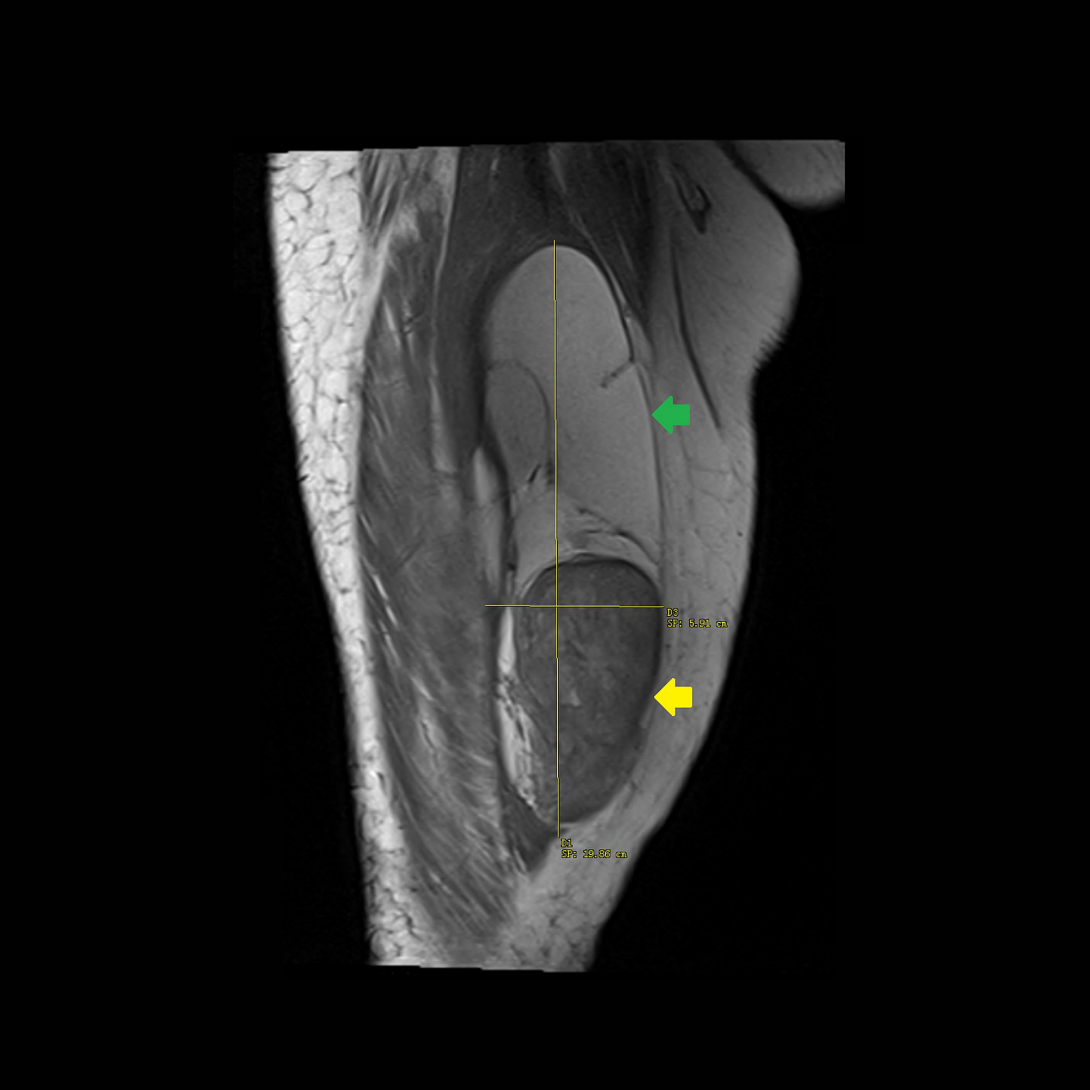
T1-weighted turbo spin echo MRI without contrast of the right thigh in coronal plane. A 20 × 6 × 5 cm multi-loculated mass with a superior lipogenic component (green arrow) and inferior non-lipogenic component measuring 9 × 5 × 5 cm (yellow arrow). MRI, magnetic resonance imaging
